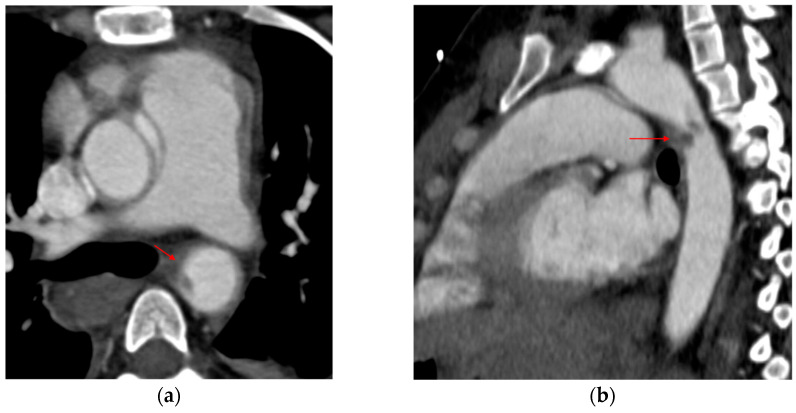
Intramural hematoma. (a) Axial and (b) sagittal CTA images demonstrate focal wall thickening (red arrows) along the anterolateral aortic wall, with an associated small intraluminal filling defect.
